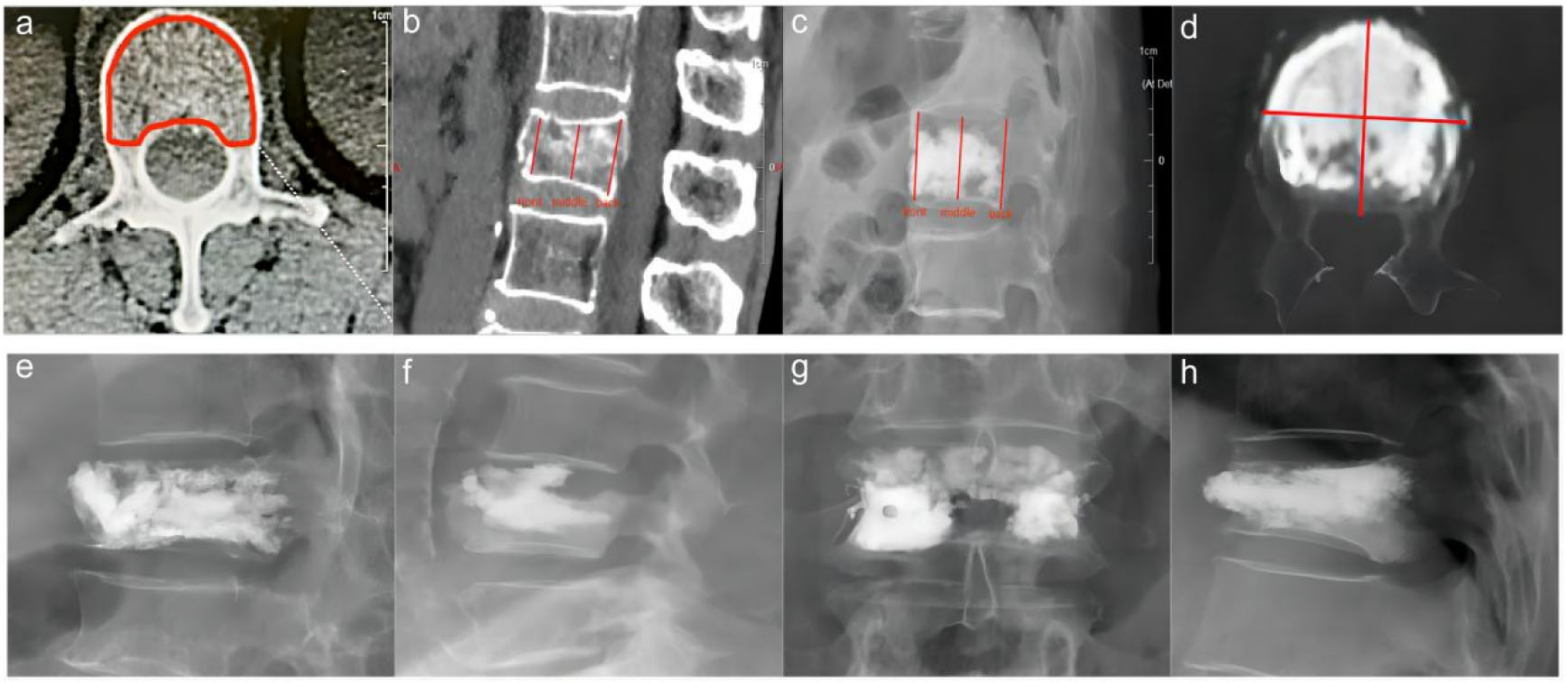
Preoperative and postoperative radiographic images of the fractured vertebra and the site of cement leakage. (A) Example of vertebral body cross-sectional area measurement. (B) Example of preoperative vertebral height measurement. (C) Example of postoperative vertebral height measurement. (D) Example of CT cross-sectional scan showing a large amount of cement distribution in all four quadrants of the vertebral body. (E) Example of bone cement leakage to the posterior vertebra; (F) Example of bone cement leakage into the intervertebral disc. (G) Example of bone cement leakage to the paravertebral vein. (H) Example of bone cement leakage to anterior vertebra.
